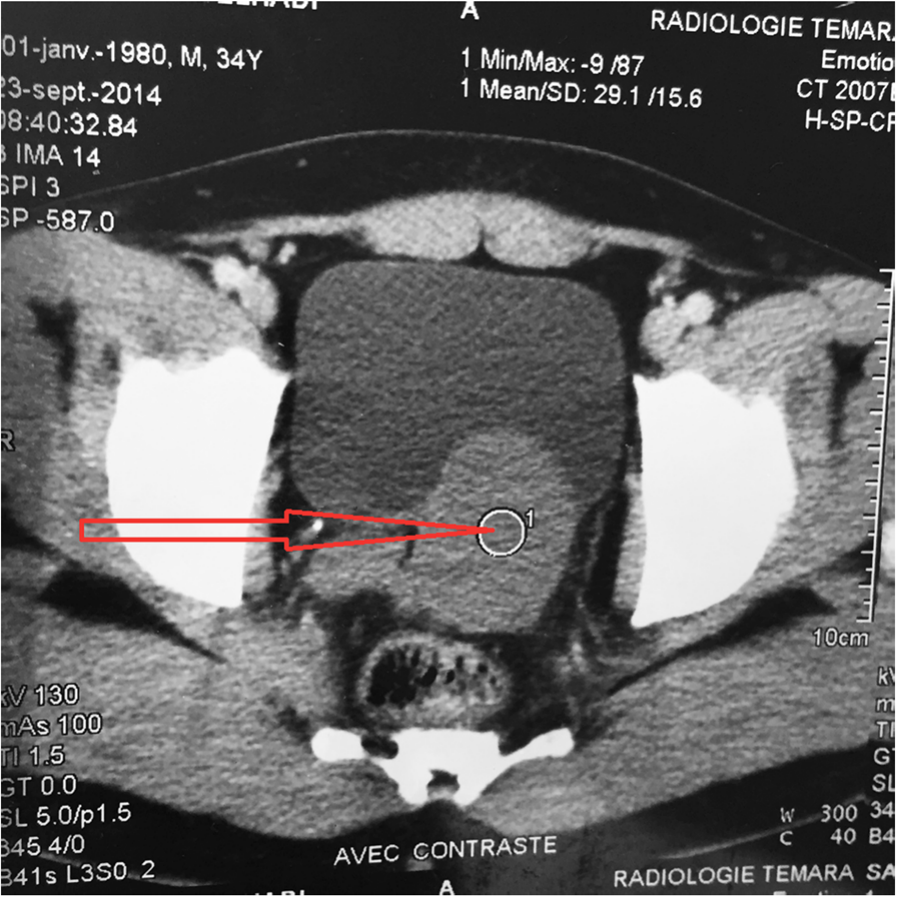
CT image showing a homogeneous liquid mass to polylobed contours not taking contrast retrovesical
